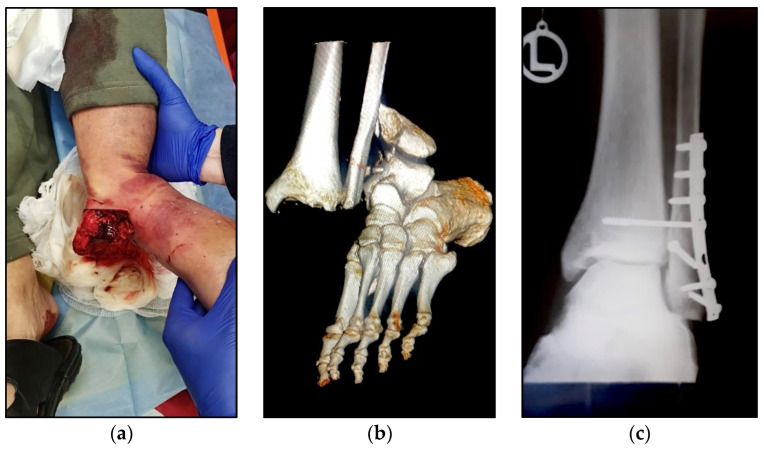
Examples of initial presentation and radiographic findings in severe ankle fractures that require talofibular trans-syndesmotic screws: (a) pre-operative aspect; (b) pre-operative computed tomography 3D reconstruction; (c) post-operative X-ray in frontal view.
